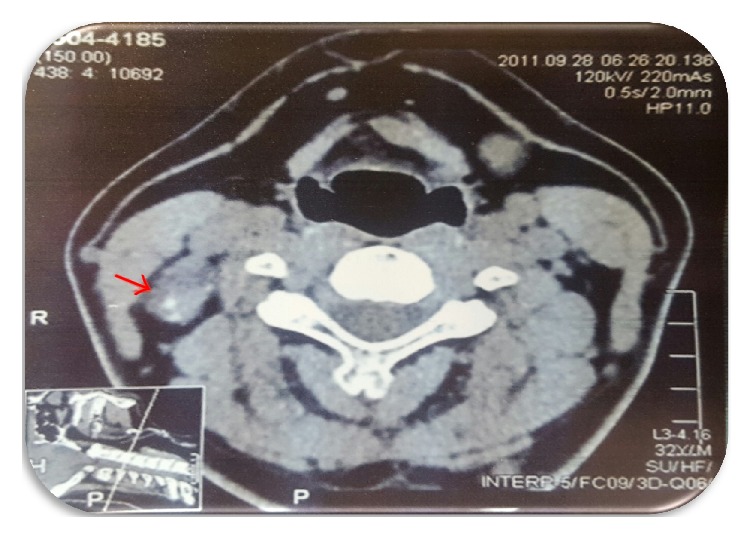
Cervical spine CT: complex right neck 2.6 cm × 2.3 cm mass incidentally noted as the posterior aspect of the jugular chain. It showed predominant decrease (20 HU) with a solid nodule (40 HU) posteriorly containing punctuate calcifications measuring 1.3 cm. Red arrow indicates right neck mass.
